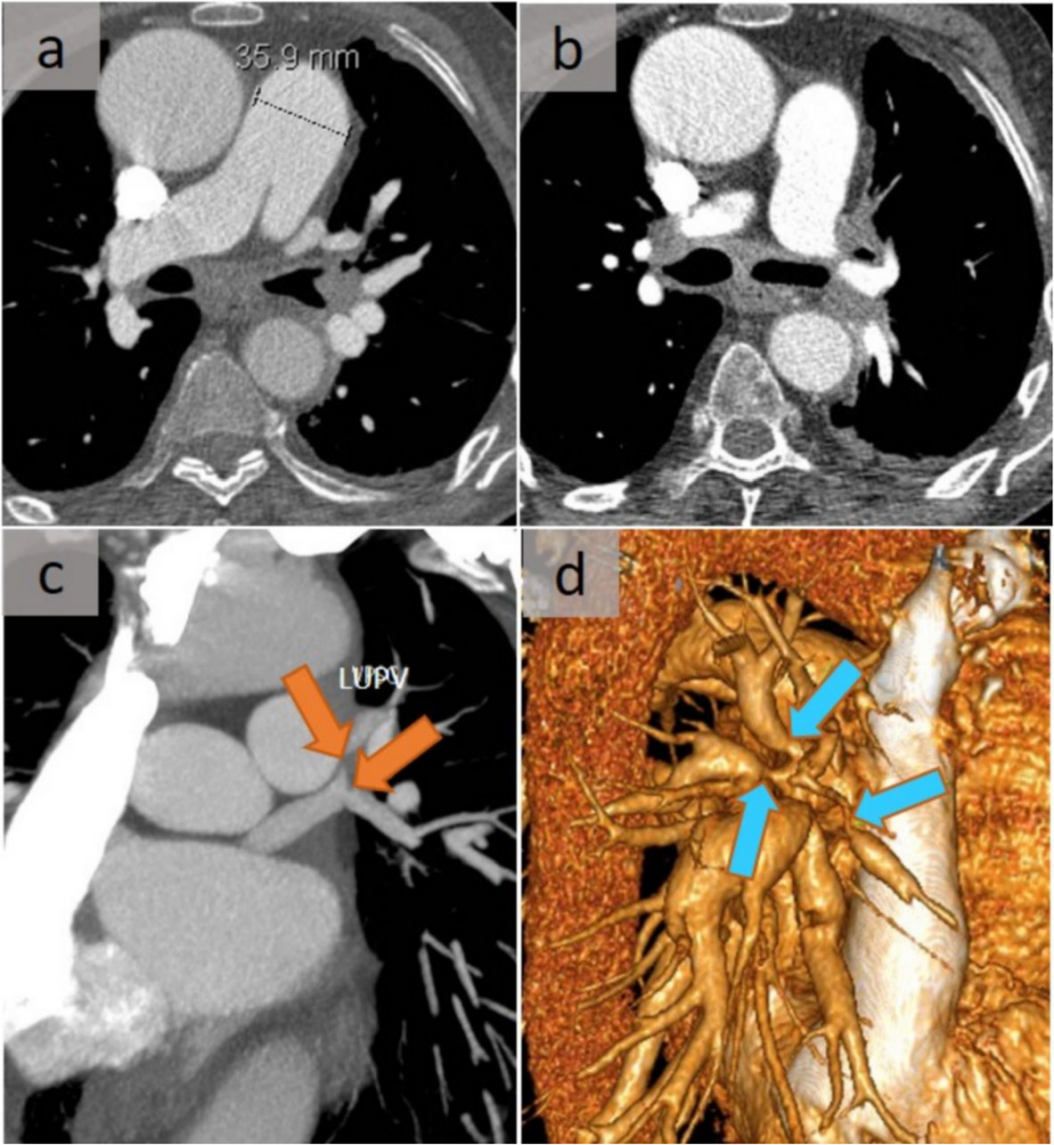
Post-mediastinitis venous complication. A patient with sclerosing mediastinitis. In addition to the encasement of the pulmonary artery, there was a narrowing of the pulmonary veins, well assessed on the successive CT slices (a, b), as well as on the oblique reformation (orange arrows) (c) and 3D view (blue arrows) (d). Diagnosis was delayed due to confounding factors related to a history of pulmonary embolism with pulmonary hypertension and tuberculosis
